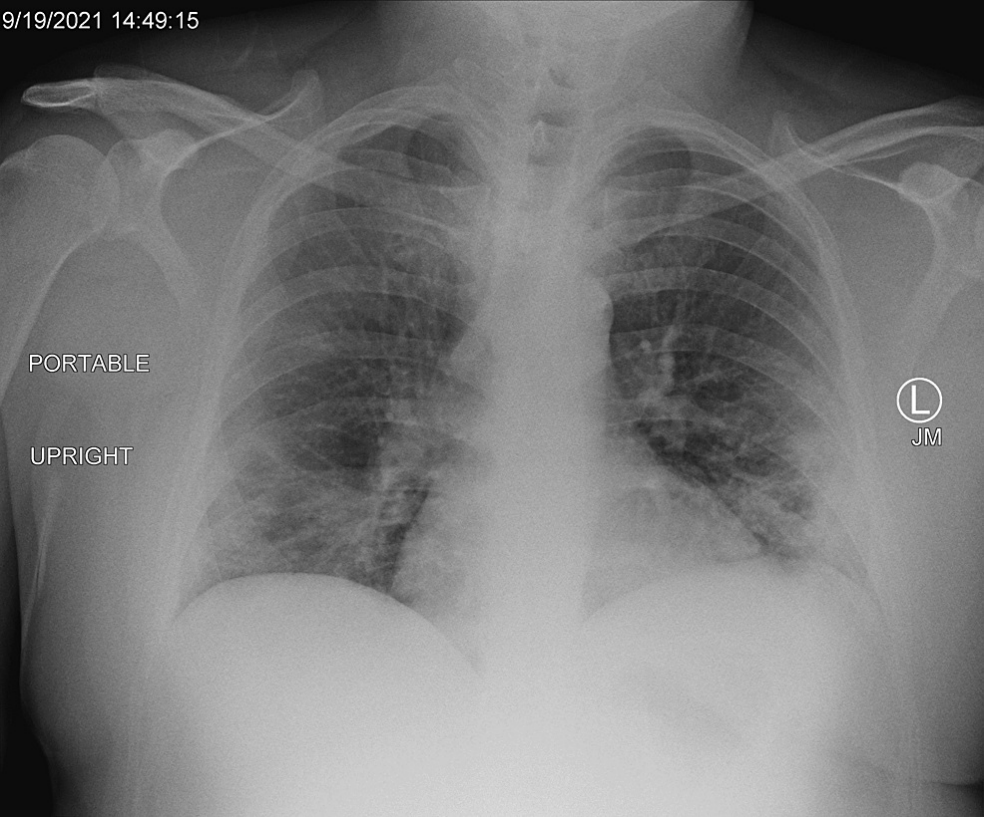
Admission chest radiograph (9/13/2021)
